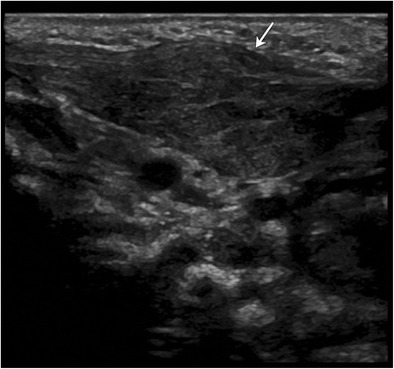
Fibromatosis colli Neck ultrasound obtained in a 6-week-old revealed fusiform enlargement of the left sternocleidomastoid without discernible underlying masses. Patient was diagnosed with fibromatosis colli. This patient had a history of dytocic birth
